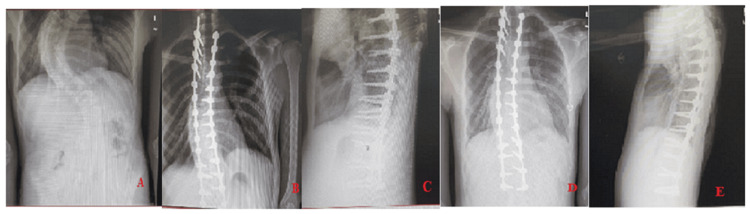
A 16-year-old male patient who underwent inferior facetectomies with pedicle screws instrumentation from T3-L4 levels. (A) preoperative posterior anterior (PA) x-rays, (B) immediate postoperative PA x-rays, (C) immediate postoperative lateral view x-rays, (D) follow-up after 12 months PA x-rays, and (E) follow-up after 12 months lateral view x-rays.
